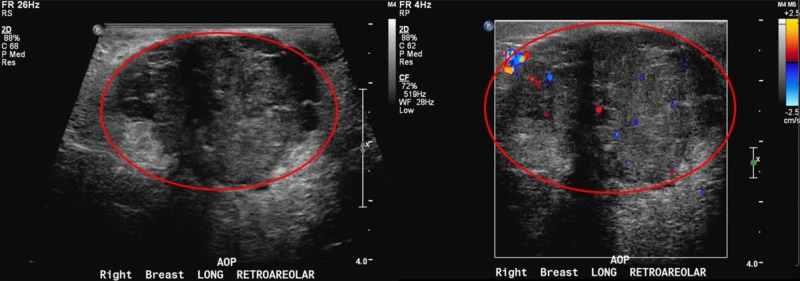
Right breast diagnostic ultrasound Right breast irregular mass with internal vascular color flow measuring 35 x 26 x 24 mm (red circle).
